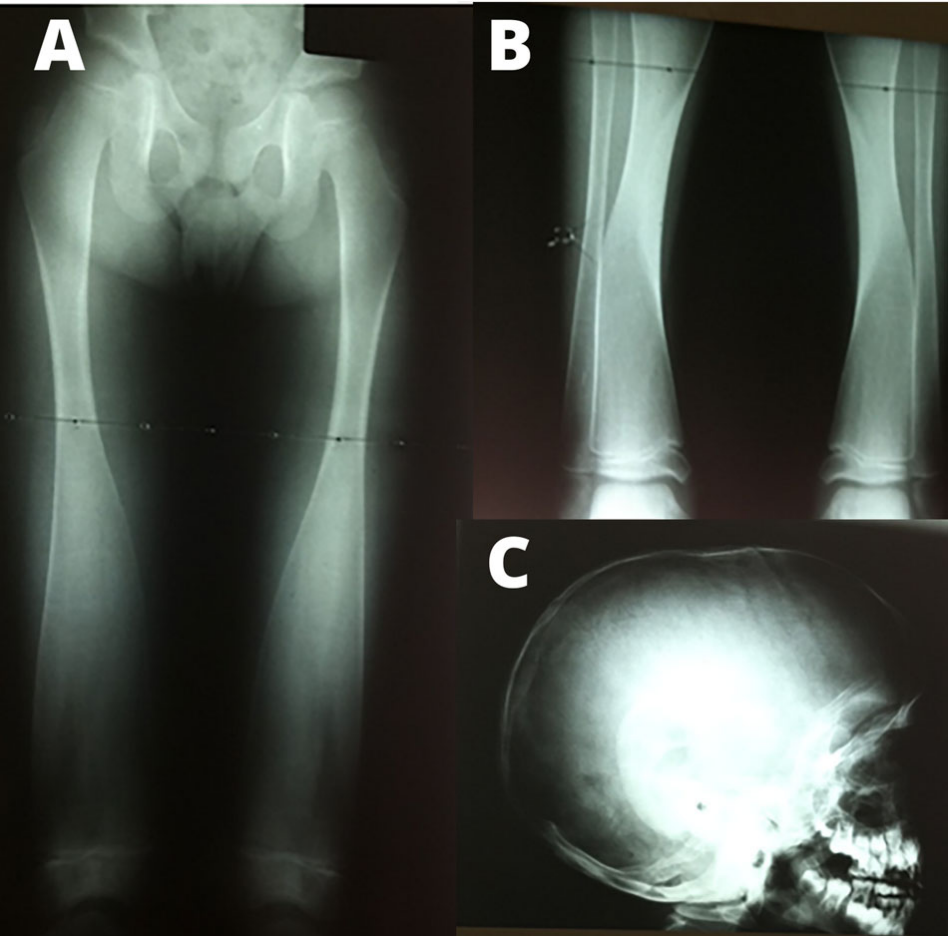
Radiographic images of a 10-year-old male with Pyle disease. A, B Erlenmeyer Flask deformity of the long bones. C Calvaria with Wormian bones.
